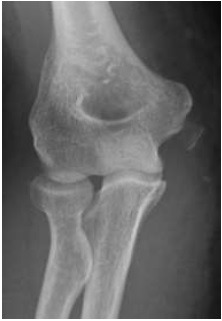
Antero-posterior and lateral radiographs after closed reduction of a simple elbow dislocation – note medial epicondyle avulsion fracture.
